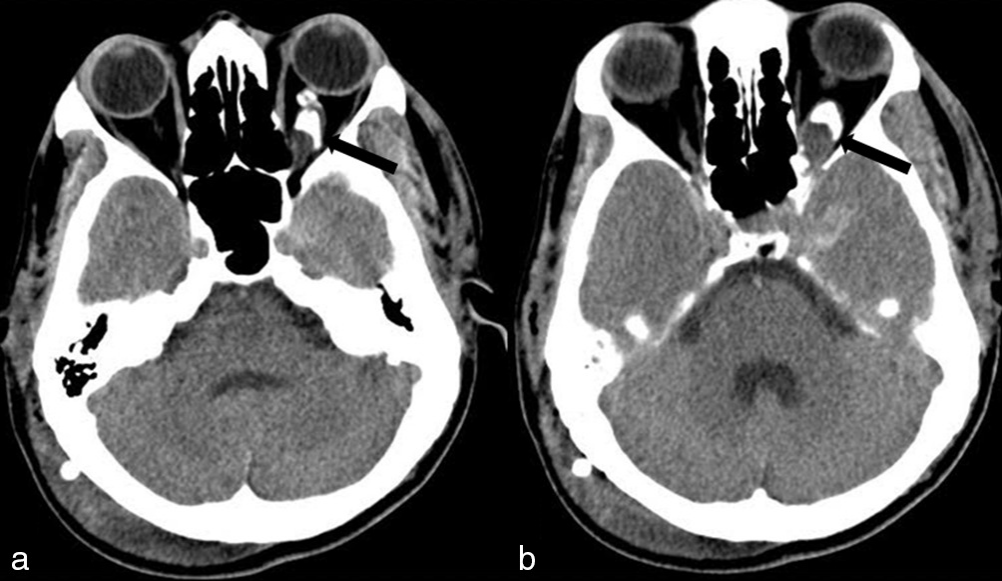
Axial non-contrast CT images. (a, b) The optic nerve sheath complex appeared thickened and tortuous and showed peripheral tram-track-like calcification with a widened optic canal (black arrows).
